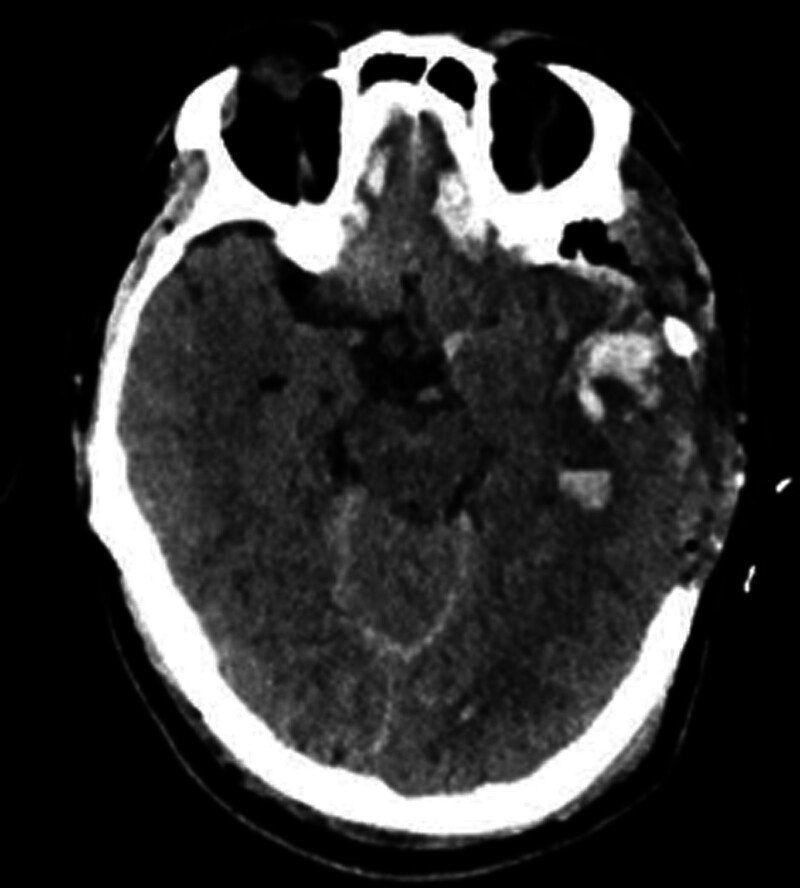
Head CT: no increase in intracranial hemorrhage. CT = computed tomography.
